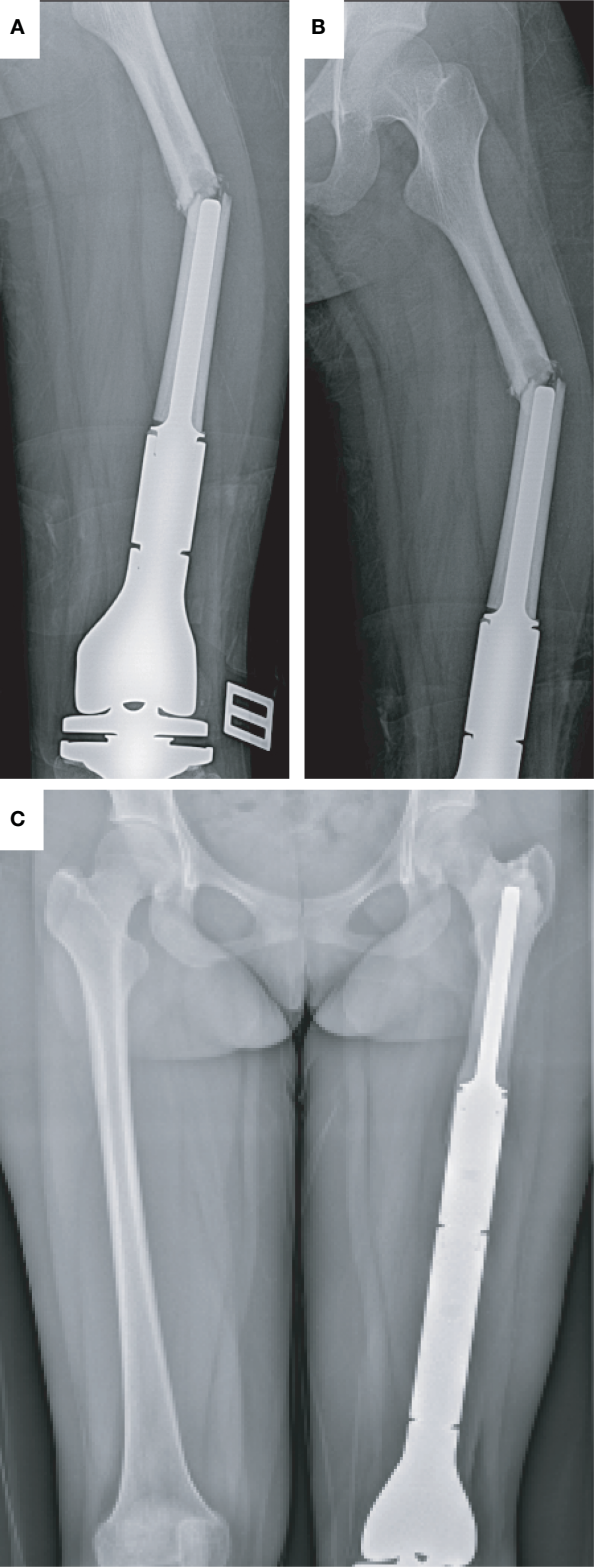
Type I periprosthetic fracture and corresponding surgical strategy (open reduction and replacement of endoprosthesis). (A, B) Preoperative X-ray image of the left femoral fracture and (C) postoperative X-ray image of the bilateral femur.
